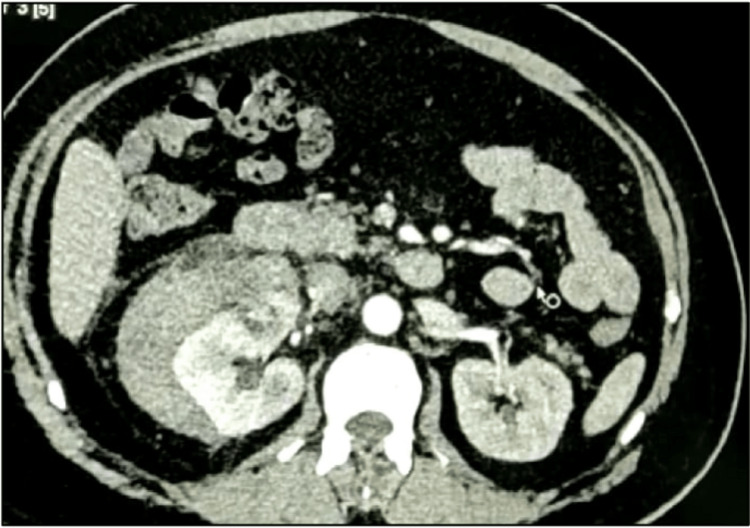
Computed tomogram showing a 2.8 cm angiomyolipoma of the right kidney
